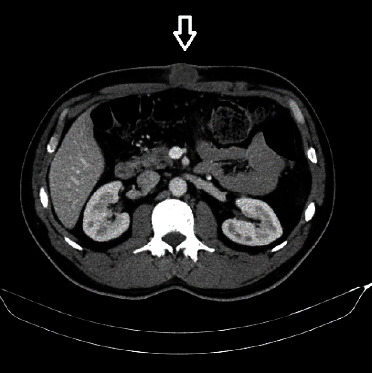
Cross-sectional computed tomography shows tumor in the abdominal wall.
